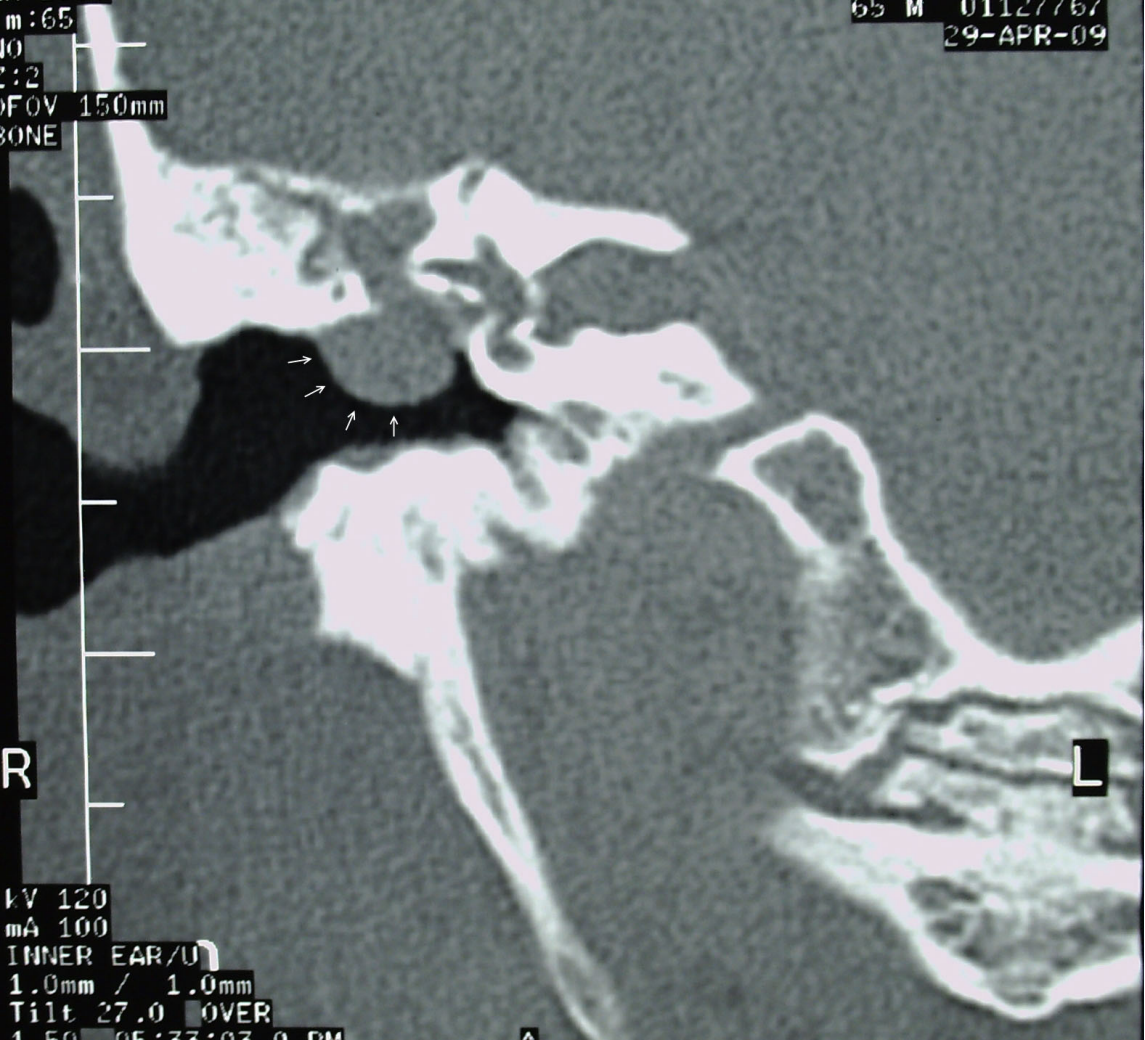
Temporal bone CT (coronal) showing a soft tissue mass occupying the mastoid antrum and part of the external ear canal. Arrows indicate the mass expanding into the external ear canal.
